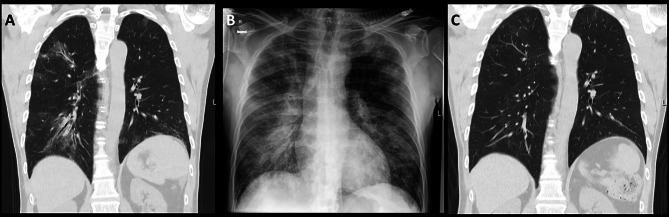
Chest computed tomography acquired on the admission day (three days before IVIG Infusion) with multifocal patchy pulmonary ground glass opacities, accentuated on the right side (A). Chest X-Ray acquired during acute respiratory deterioration (B) with progressive bilateral opacifications, consistent with non-cardiac pulmonary edema. Chest computed tomography acquired 2 months after discharge from the hospital with almost complete regression of the ground-glass opacities (C)
